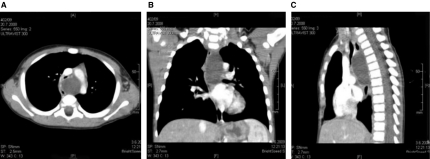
Contrast-enhanced computed tomography (CECT) of the thorax with multiplanar reconstructions. A: axial scan;B: coronal scan;C: sagittal scan.
